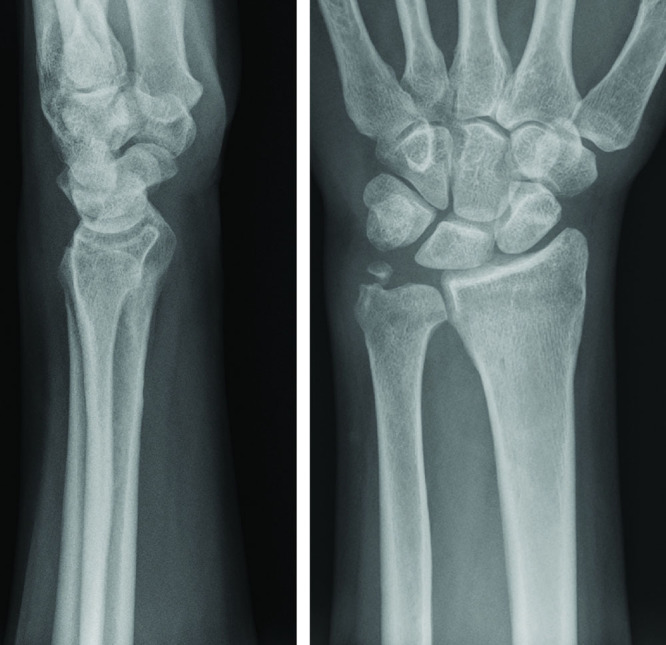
Antero-posterior and lateral X-ray follow-up examinations revealing the correct reduction and function of the RUD.
